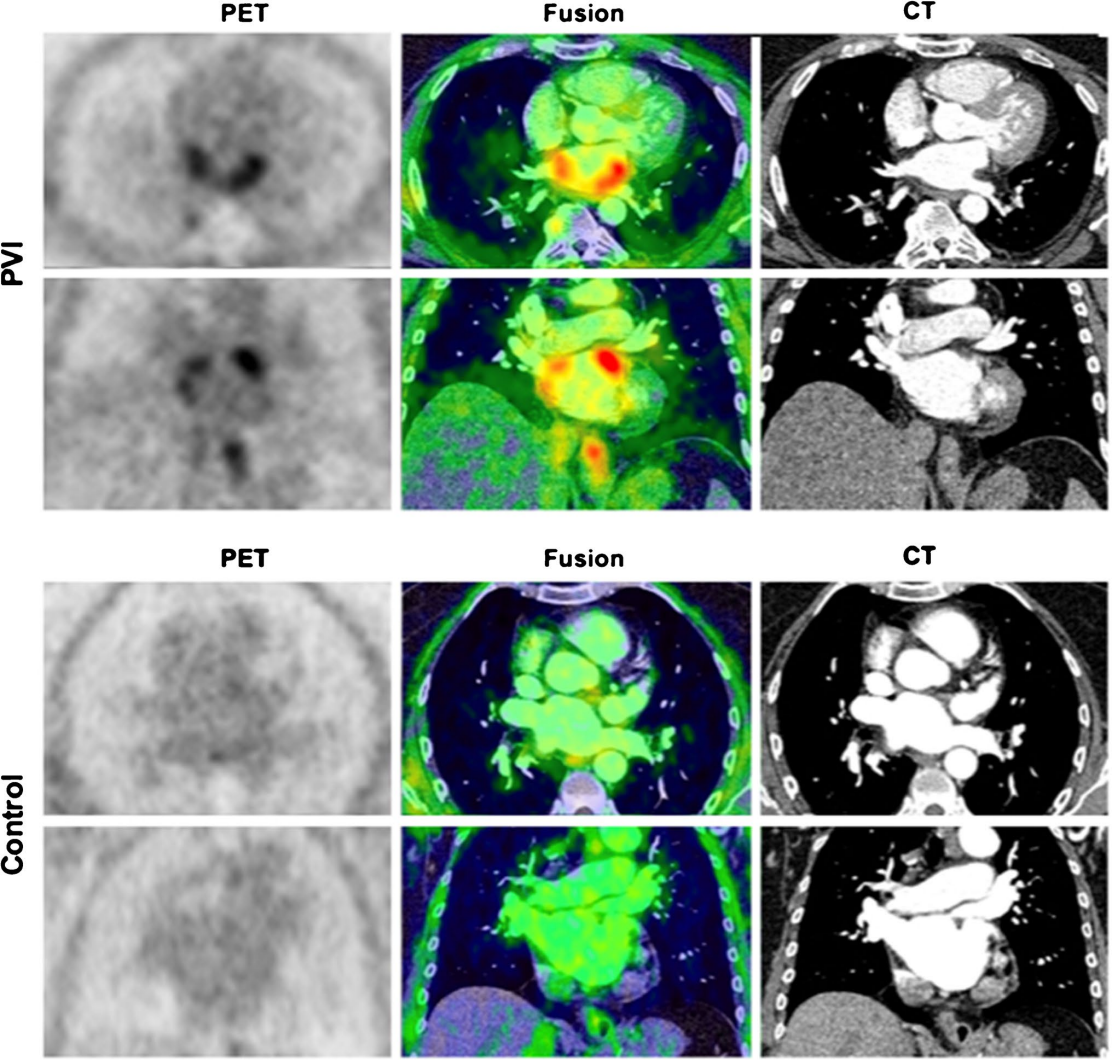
Localized FAPI uptake in a PVI patient vs. control. Upper row (PVI): example of a distinct focal visual uptake in PV antra after PVI; lower row (control): no visual uptake in a patient having undergone PET imaging for tumor staging; PET (left panel), PET/CT fusion (middle panel) and CT (right panel). PV, pulmonary veins; PVI, pulmonary vein isolation; PET, positron emission tomography; CT, computed tomography
